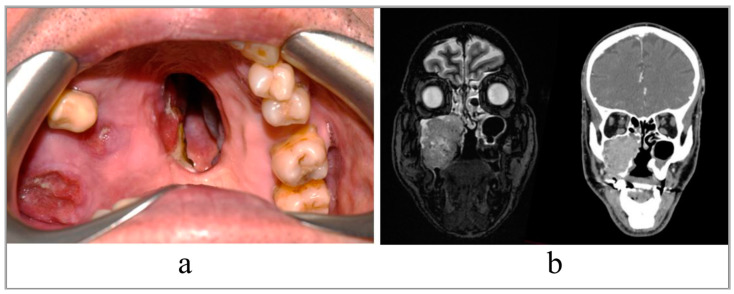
(a) Intraoral view of the tumor; (b) preoperative RMN and CT scan, coronal slices.
